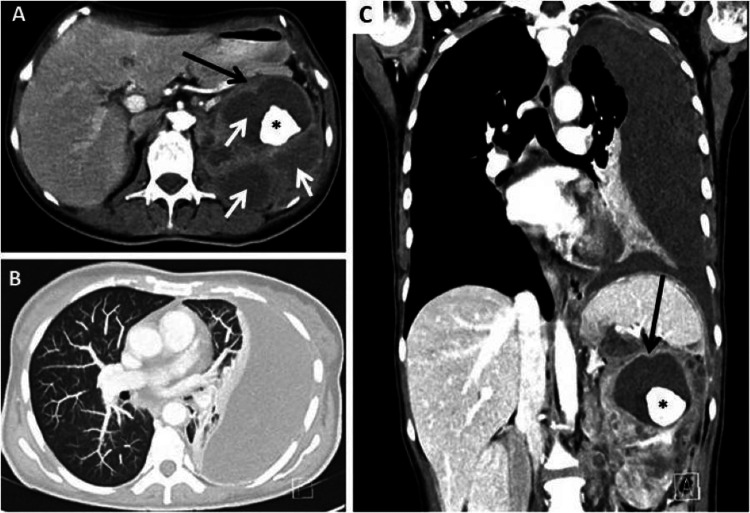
Thoracic and abdominal CT Images show a structural alteration of the left kidney with almost complete substitution of the parenchyma by cystic lesions, with dimensions varying from millimeters to 7 centimeters (Figures 1A, 1C; black arrow), some of these with gross intraluminal calcifications and a staghorn calculus (Figures 1A, 1C; asterisk). Delayed uptake of contrast in the affected kidney was documented reflecting hypofunction of the renal parenchyma. Several hypoattenuating round areas throughout the enlarged kidney resembling the paw print of a bear (bear paw sign) can be seen in Figure 1A (white arrows). In Figures 1B, 1C, a large pleural effusion with passive atelectasis of left inferior lobe can be seen.
